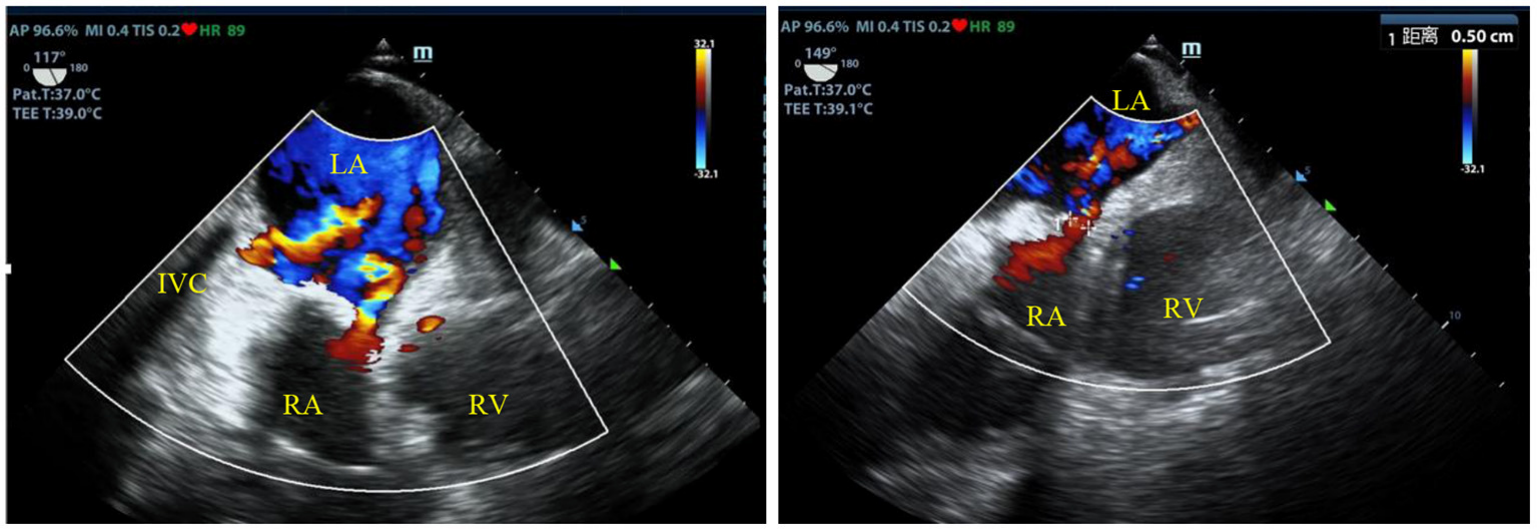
TEE showed a residual atrial septal defect with a size of 5 mm and bidirectional multicolored shunt flow signal. And an IVC-left atrium shunt was also found soon after the repair. TEE, transesophageal echocardiography; LA, left atrium; RA, right atrium; RV, right ventricle; IVC, inferior vena cava.
